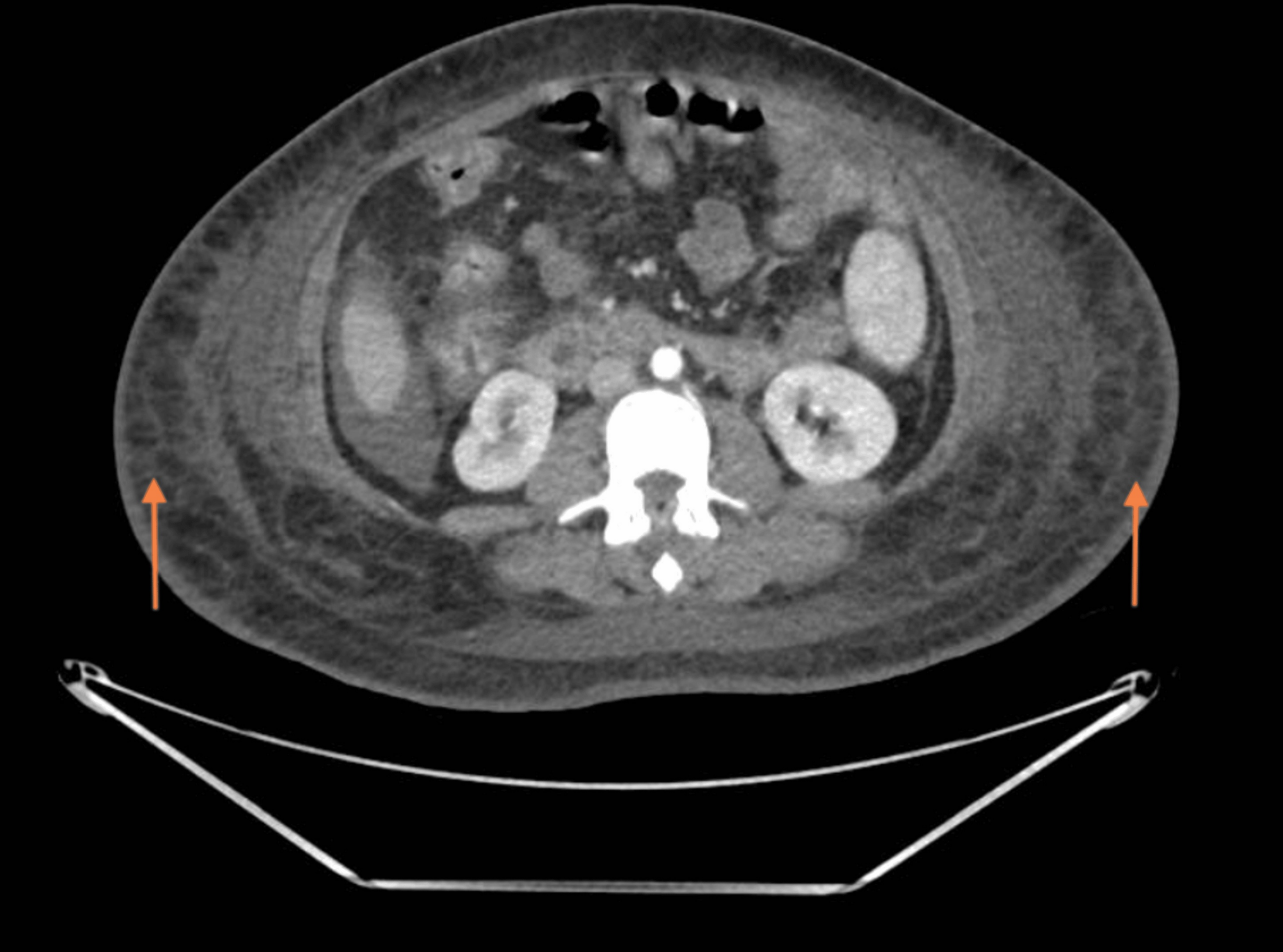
CT abdomen cross slice from CT chest abdomen and pelvis. Significant bilateral subcutaneous oedema delineated by arrows.
